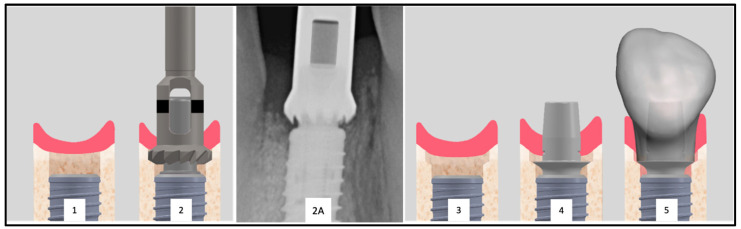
Inserted implant with clearly visible proximal bone overhangs (1). To allow the placement of the provisional restoration a bone profiler is used (2). Radiographic image of an exemplary Bone Profiler (2A). This tool reduces the bone of the supracrestal complex according to the diameter of the abutment (2). The conditioned bone is visible (3). The conditioning of the bone enables the seamless placement of the abutment (4) and the restoration (5).
